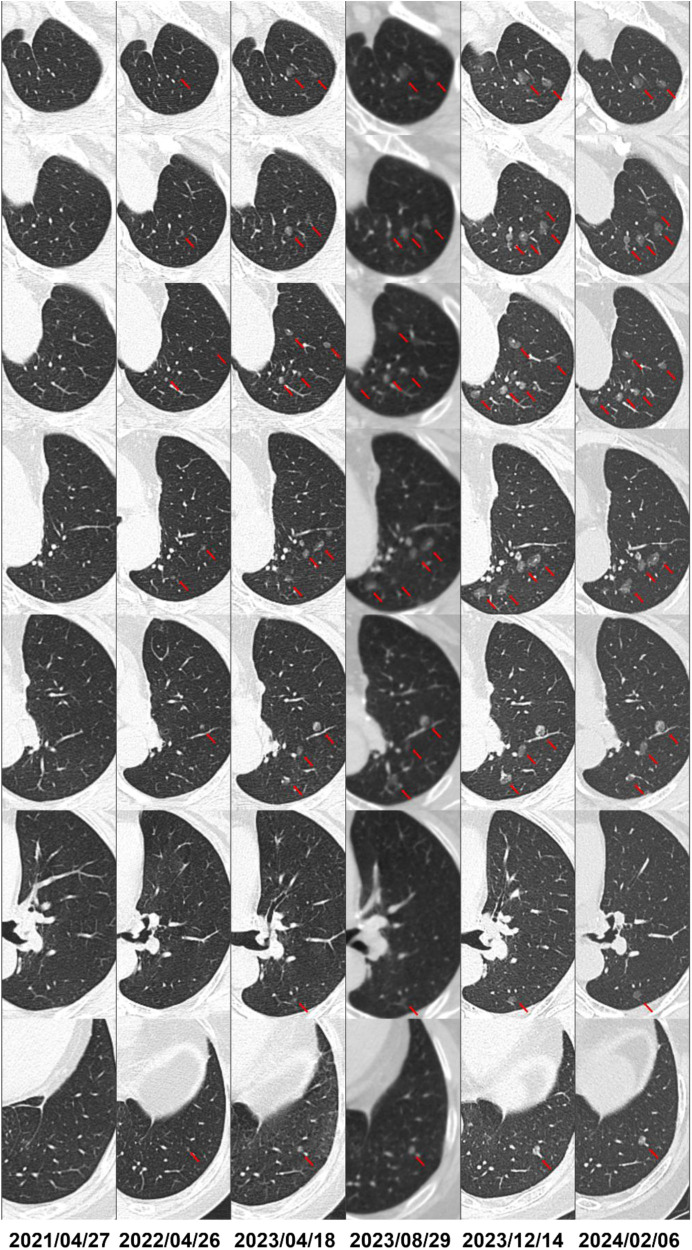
Follow-up chest CT images demonstrating the evolution of lesions in the left lower lobe. The scan on April 27, 2021, showed no abnormalities. By April 26, 2022, tiny lesions became apparent. Subsequent imaging revealed that the multiple GGNs in the left lower lobe had significantly increased in size compared to earlier examinations.
